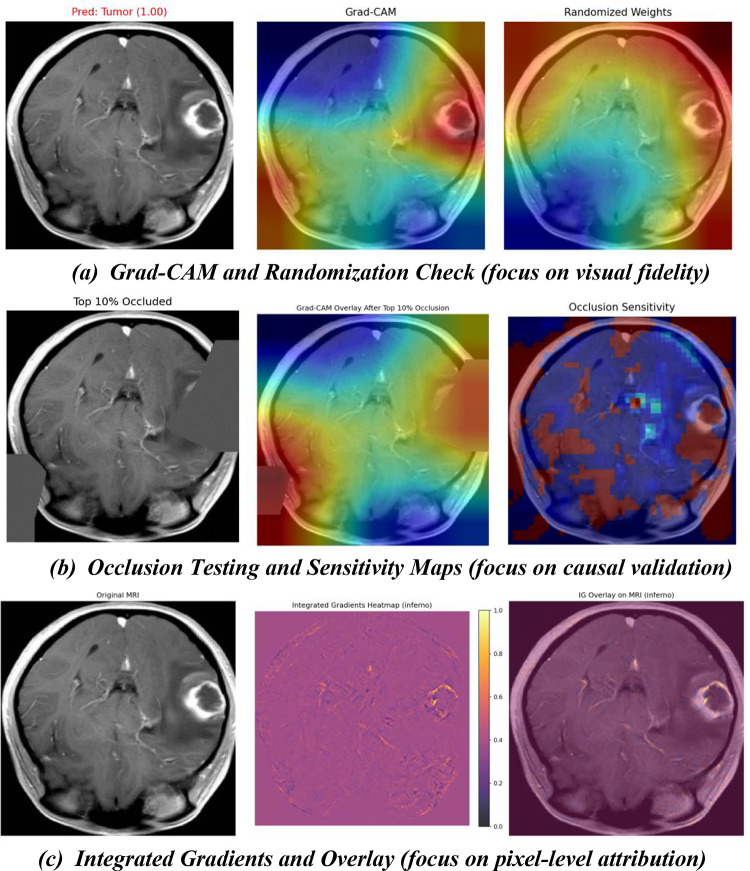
Representative Example of XAI Methods on Brain MRI Tumor Case from External Dataset. (a) Grad-CAM highlights tumor regions, while randomization disrupts activations. (b) Occlusion testing and sensitivity maps confirm causal relevance of highlighted areas. (c) Integrated Gradients overlays, visualized with a perceptually uniform colormap (Inferno), provide pixel-level attribution consistent with Grad-CAM, reinforcing interpretability reliability.
